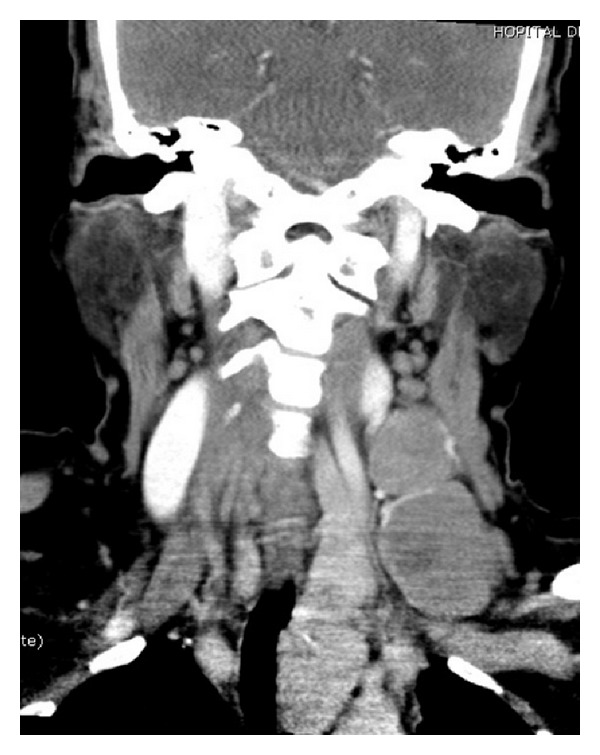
Contrast enhanced neck CT scan coronal cup: heterogenic left lateral tracheal tissue mass, probably a left diving thyroid nodule with 2 voluminous left lymphadenopathies with heterogenic density and no calcifications.
